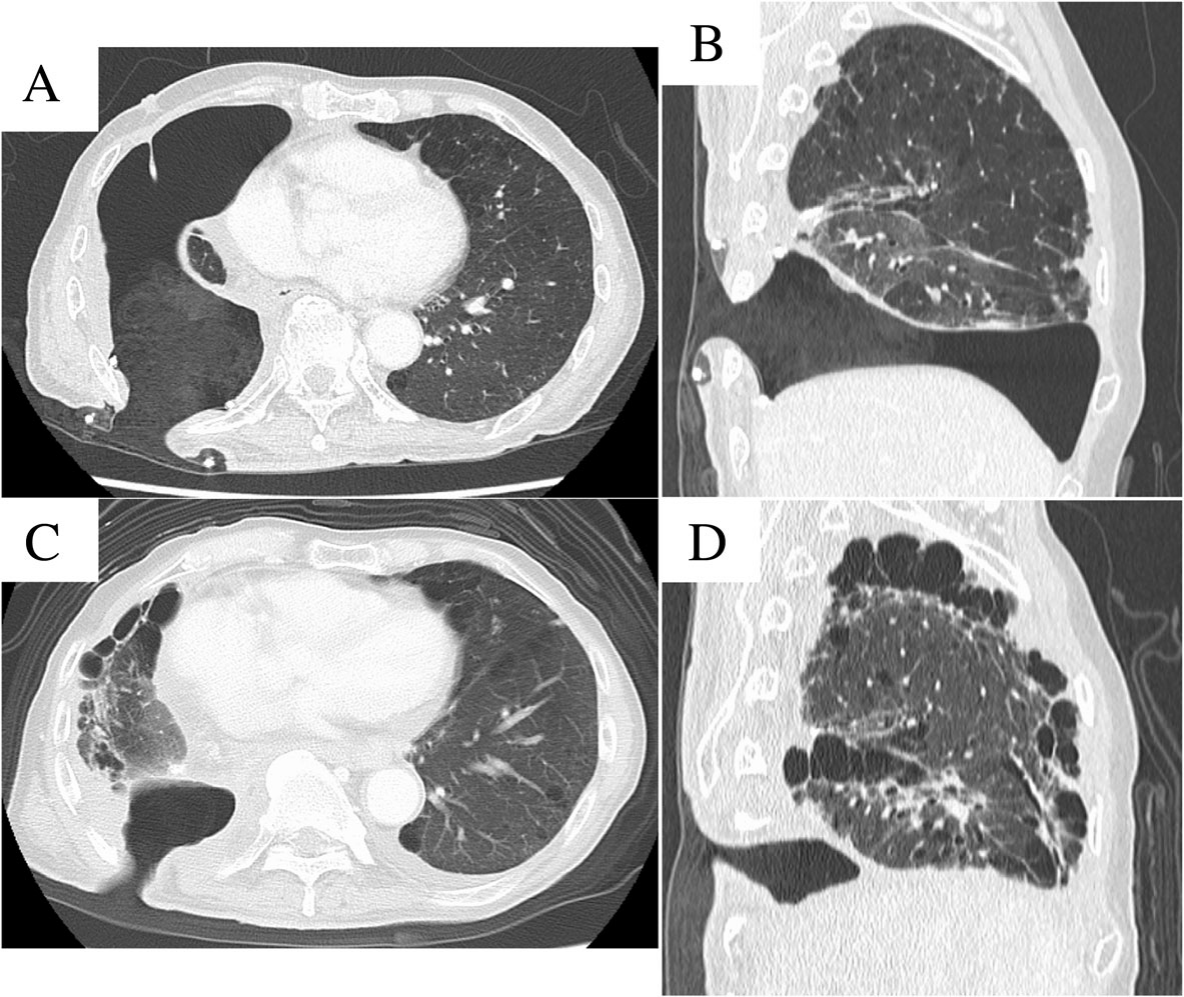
The CT shows the change of the thoracic cavity after fenestration surgery. The image obtained 1-month post-procedure (a axial view, b sagittal view). Image obtained 1-year post-procedure (c axial view, d sagittal view)
